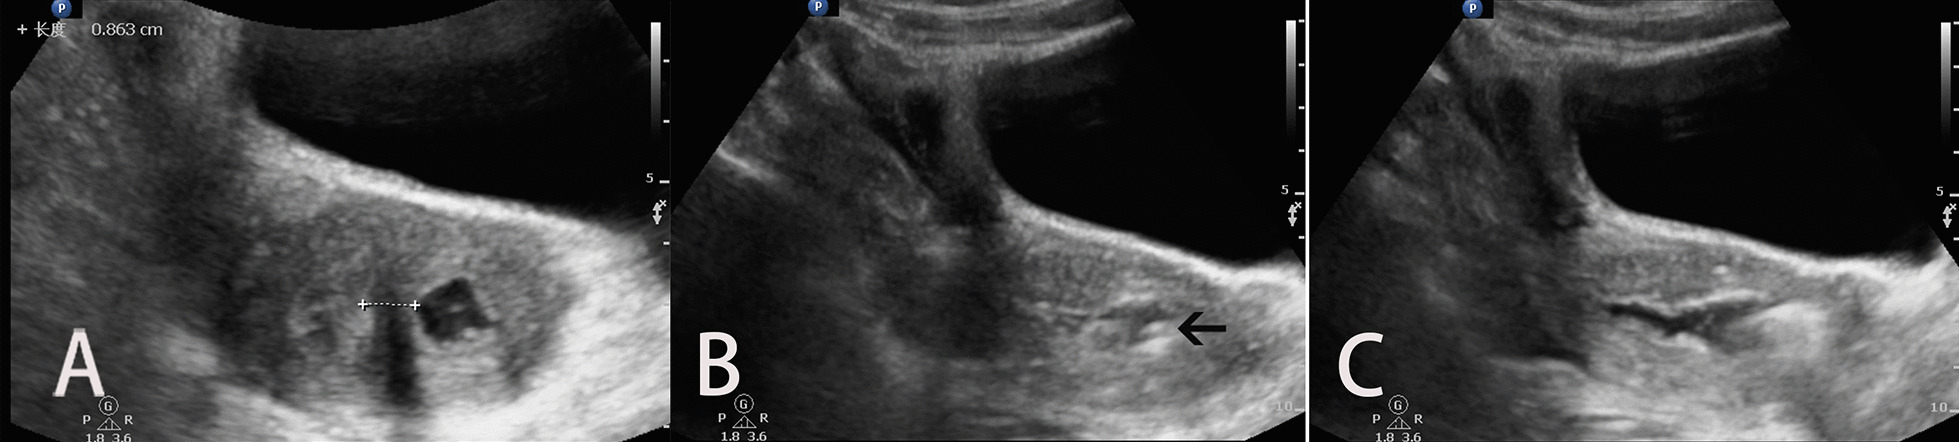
Ultrasonic monitoring picture A Only the filling of the left uterine cavity could be seen on the ultrasound, and the asymmetric uterine septum could be clearly shown and measured in this section. B Real-time display of electric hook position under bladder filling (black arrow). C Abdominal ultrasound monitoring of uterine cavity communication
